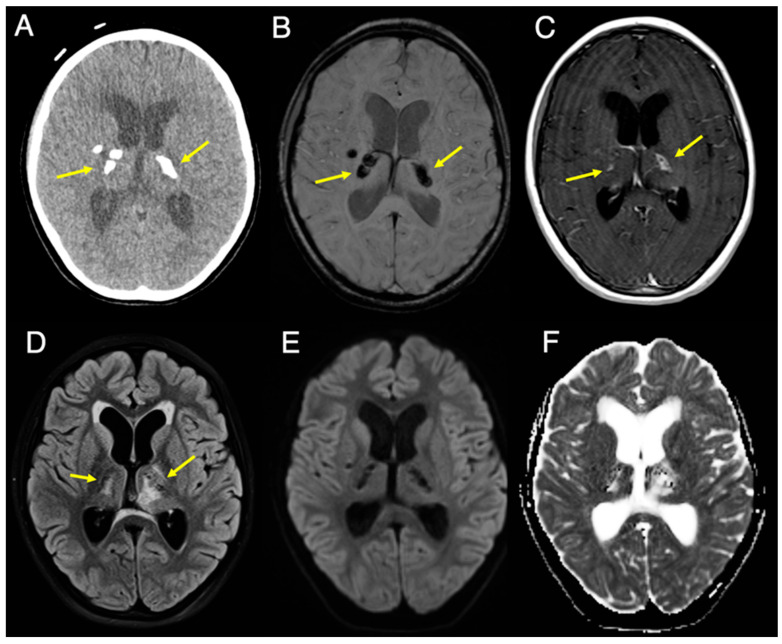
Axial CT and 3T MRI slices at the level of the basal ganglia. (A) Same-day CT image (brain window) and (B) SWI image shows bilateral coarse basal ganglia calcifications, primarily involving the thalami (arrows). (C) Contrast-enhanced T1-TSE image shows mild peripheral enhancement surrounding the calcifications (arrows). (D) Fat-saturated FLAIR image shows peripheral T2 hyperintensities (arrows) surrounding the calcifications, similar to those seen in the pons in Figure 1. (E,F) DWI and ADC map show no associated diffusion restriction.
